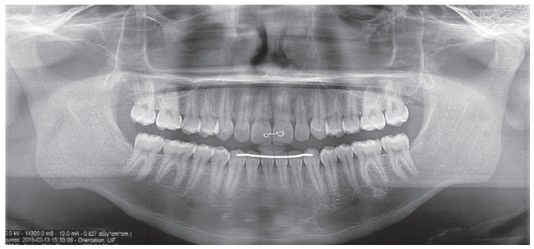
Follow-up panoramic radiograph, 5 years after orthodontic treatment.
